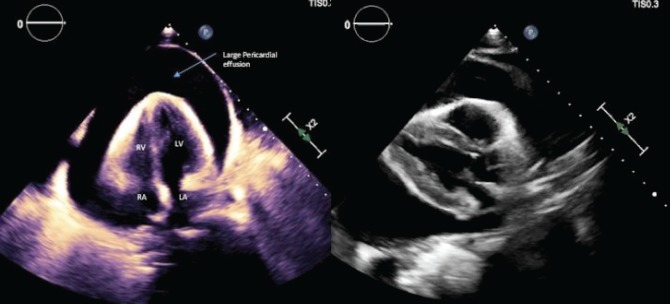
(a) Transthoracic echocardiogram, subcoastal view with a large pericardial effusion. (b) Transthoracic echocardiogram, parasternal long-axis view demonstrating a large pericardial effusion.
